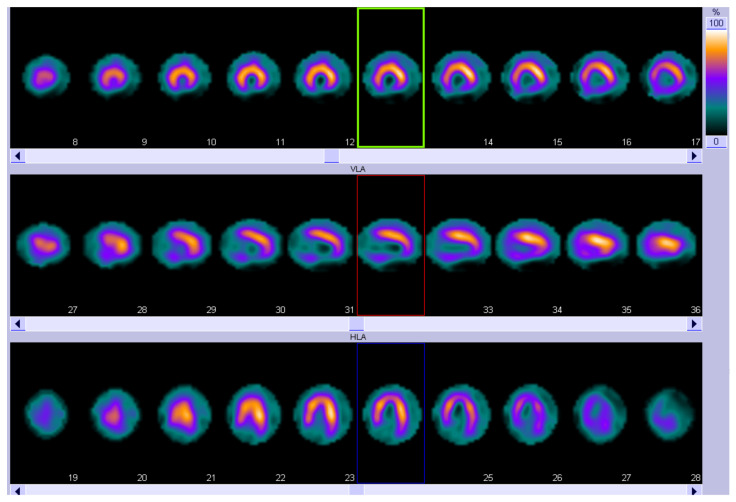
^123^I-MIBG SPECT showing an extensive area of reduced innervation in the inferior apex and inferior wall of the left ventricle. Short-axis view (top row), vertical long-axis view (middle row), and horizontal long-axis view (bottom row). MIBG, Meta-iodobenzylguanidine; SPECT, Single Photon Emission Computed Tomography.
